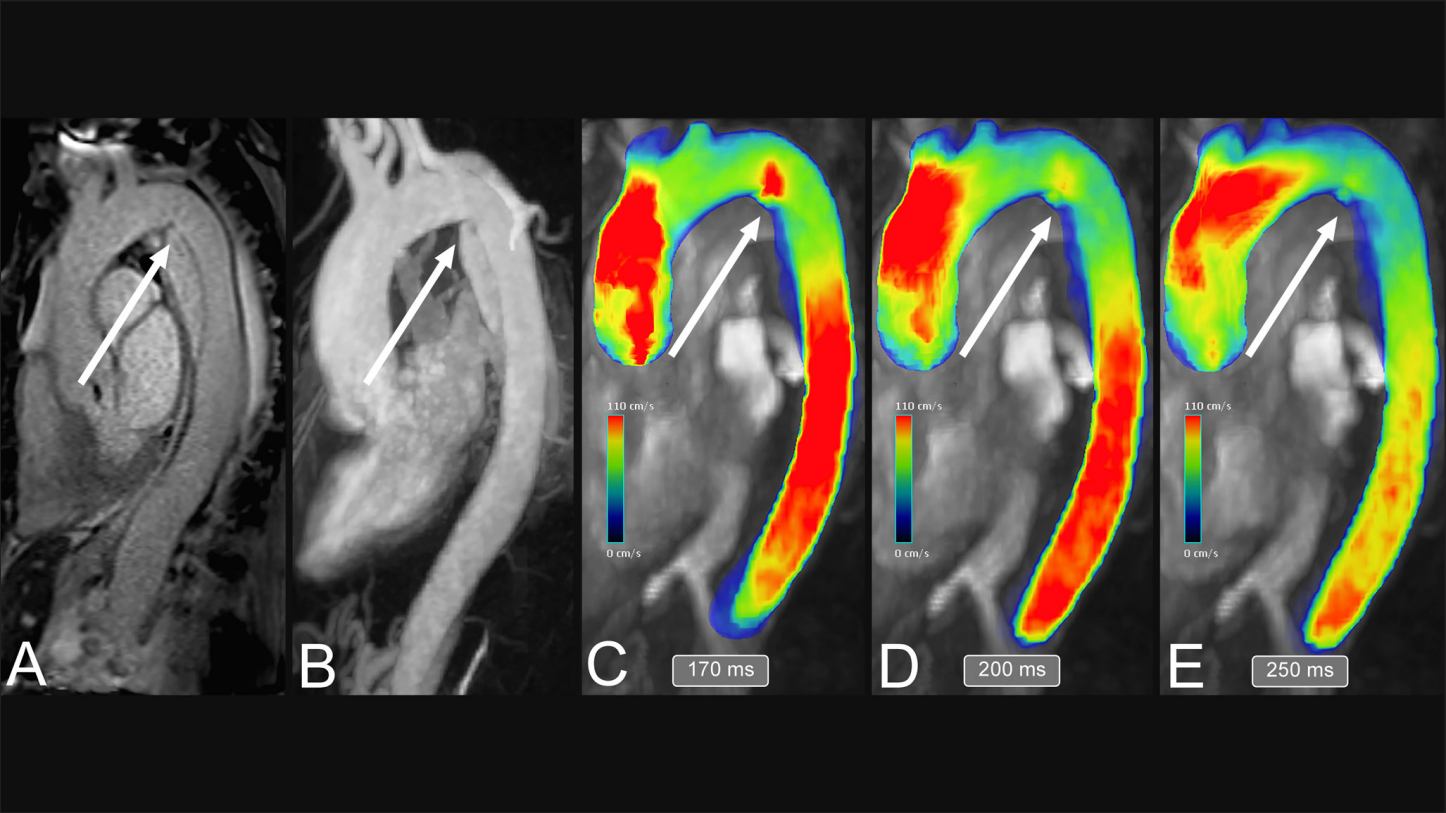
Qualitative analysis of type-B aortic dissection using magnetic resonance imaging (MRI). The contrast-enhanced MRA sequence (A) gives information on the site of the primary entry tear (white arrows), while the TWIST sequence shows the intimal flap and the directionality of false lumen filling (B). 4D flow MRI sequence (C-E) may complete the assessment of aortic dissection with color-coded velocity mapping, which is potentially able to uncover the tear where the false lumen is filling through (white arrows, C-E).
